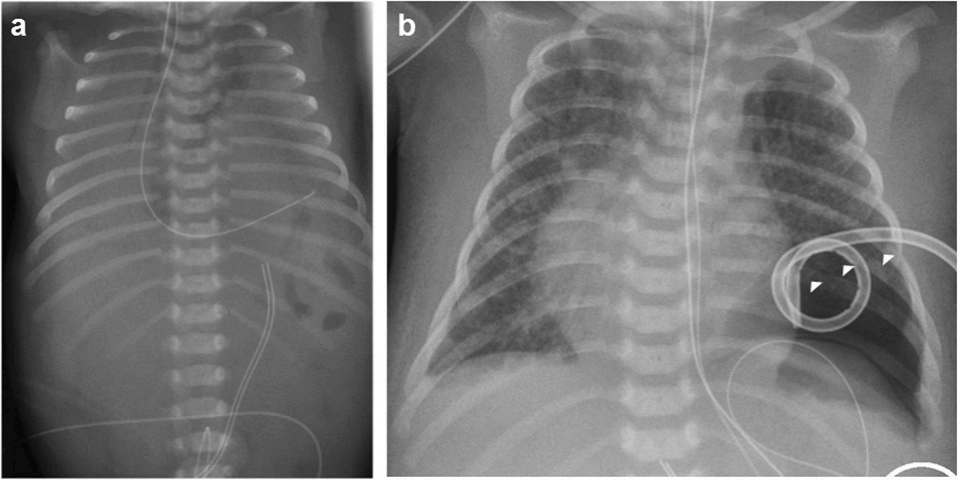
Preoperative (a) and postoperative x-rays (b) of the index patient. Arrowheads mark the pneumothorax in (b).
